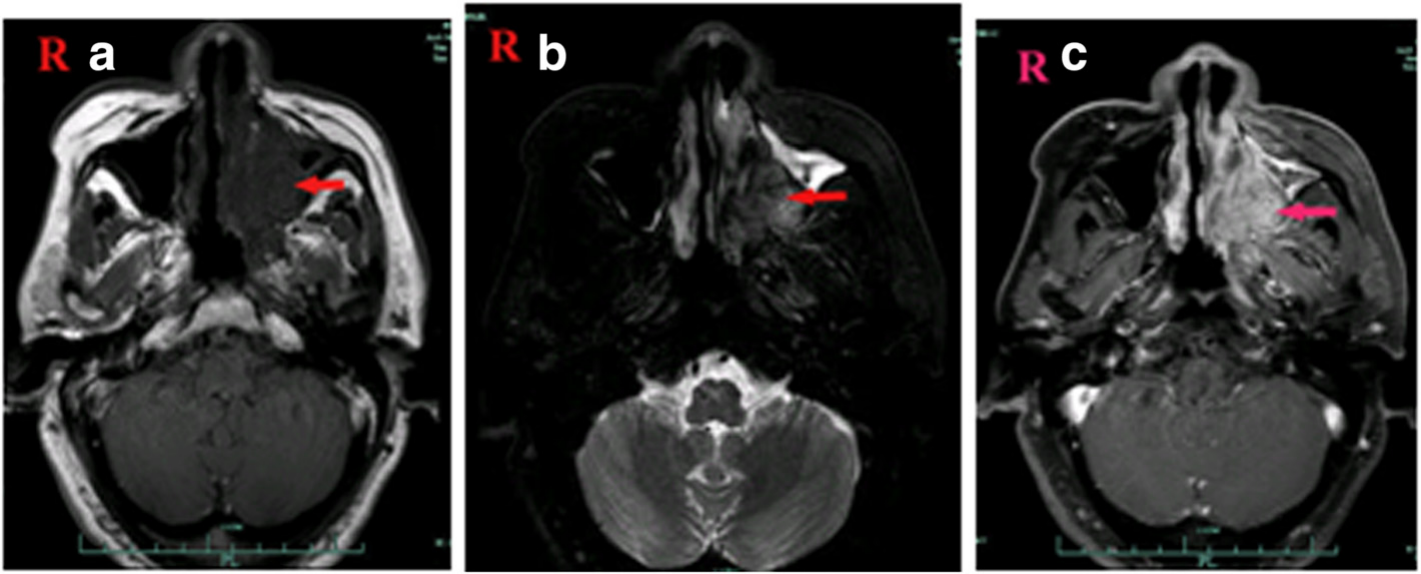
MRI of Case 2 showingan abnormal signal in the left maxillary sinus, left nasal cavity, and nasopharynx. a) TheT1-weighted signals were hypointense, b) the T2-weighted signals were hyperintense, c) and the contrast-enhanced T1-weighted MRI images show strong enhancement.
